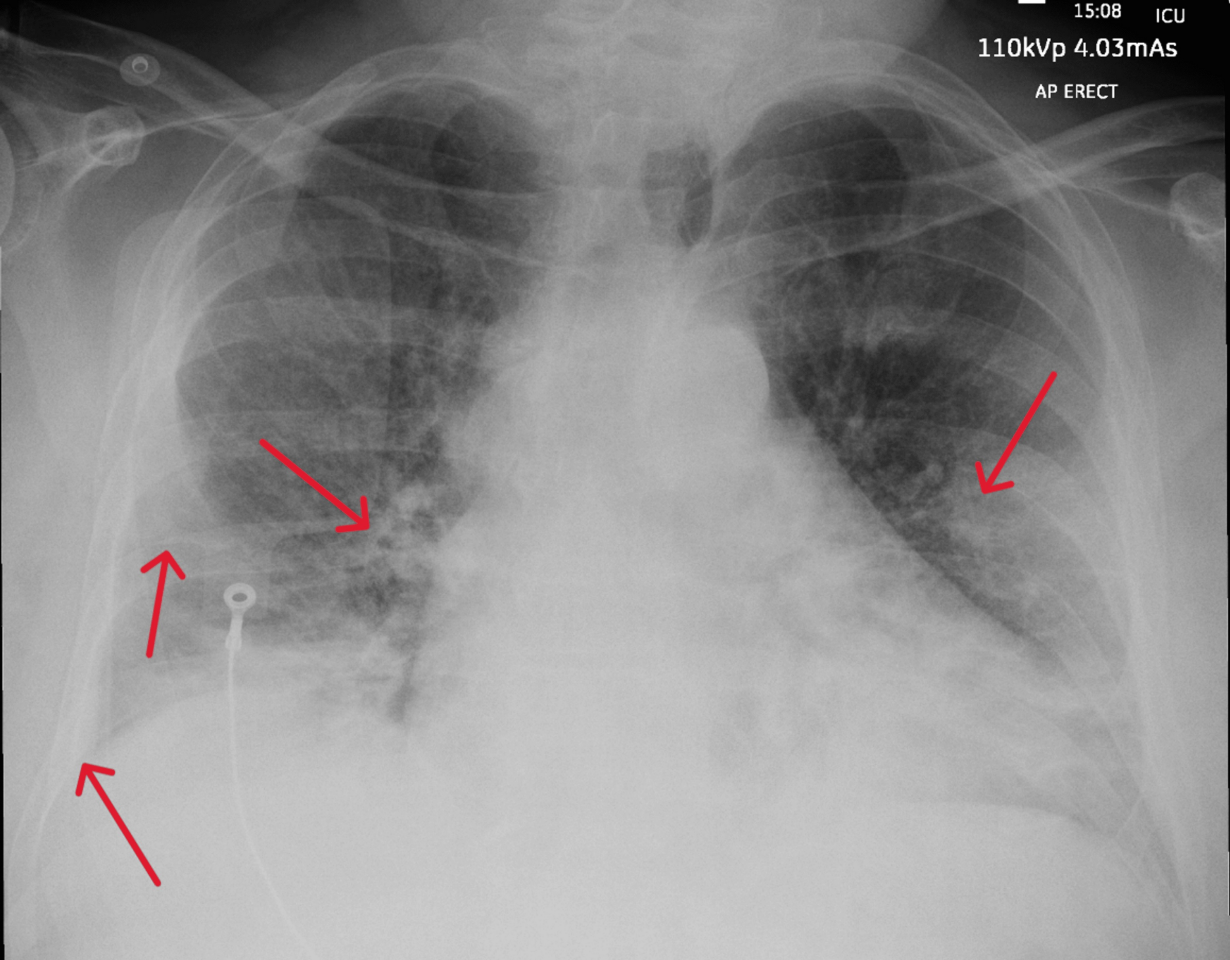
Admission chest X-ray demonstrating evidence of pulmonary congestion and infiltrates (marked with red arrows)
